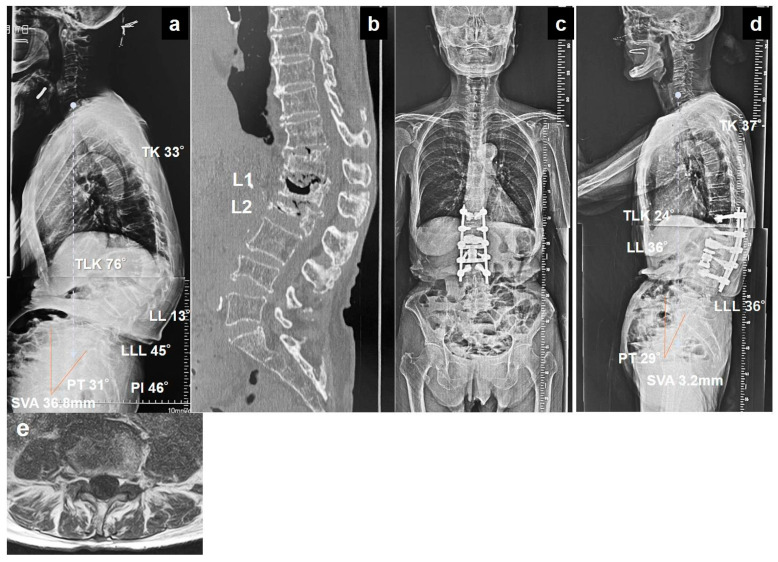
Short fixation with CAPS without revision surgery (Case 3). Preoperative standing lateral radiograph (a) and CT (b) demonstrating kyphotic deformity due to severe wedge deformity at L2 and nonunion at L1. The patient maintained compensated global sagittal alignment (SVA, 36.8 mm) with pelvic retroversion (PT, 31°) and lower lumbar lordosis (45°). Postoperative radiographs after staged kyphoplasty, L1 corpectomy, and posterior percutaneous pedicle screw fixation using cement-augmented pedicle screws demonstrate restoration of satisfactory sagittal alignment without the need for pelvic fixation (c,d). Axial T1-weighted MRI at the L4–L5 demonstrates mild fatty degeneration of the paraspinal muscles, corresponding to Goutallier grade 2 (e).
